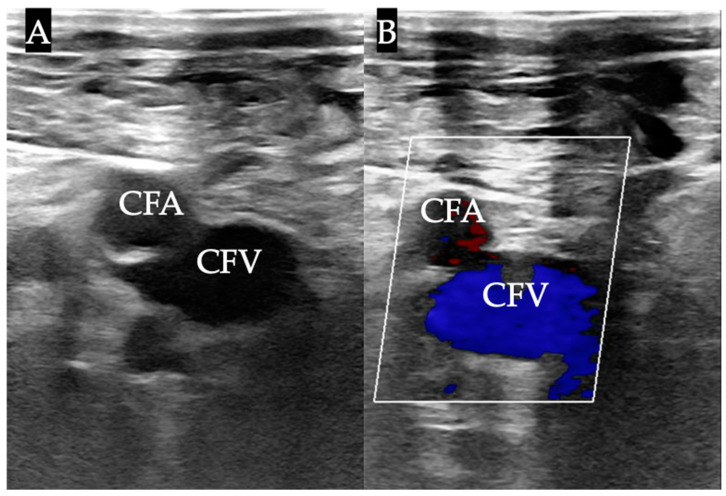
Example of a two-dimensional ultrasound transversal view of the groin vessels with and without color Doppler. Ultrasound images demonstrating a transversal view of the right groin, showing the femoral vessels. The femoral artery and vein are visualized in a transverse plane without (A) and with color Doppler ((B), artery: dark red color, vein: blue color), providing clear differentiation between the vascular structures. CFA, common femoral artery; CFV, common femoral vein.
